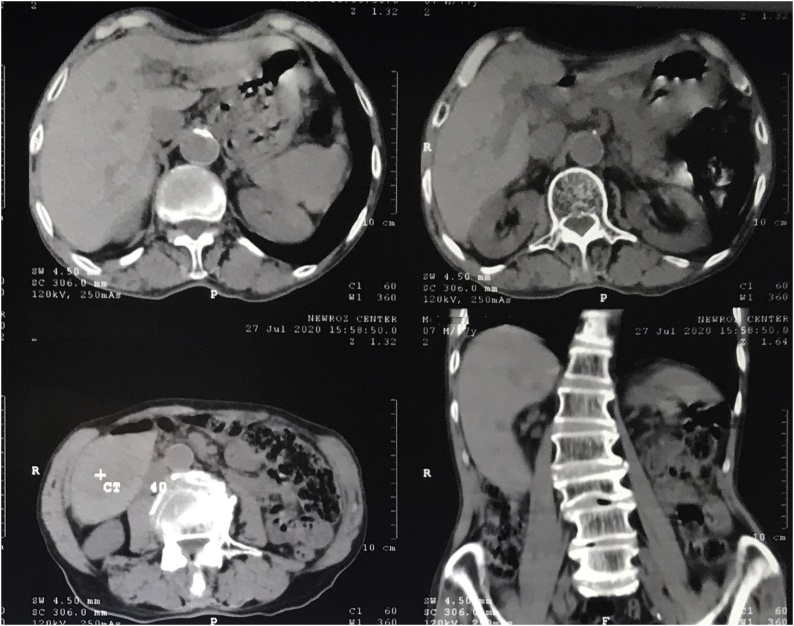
Native CT-scan of the abdomen showing liver tumor in segment VI, the gall bladder is not visualized in the films.
